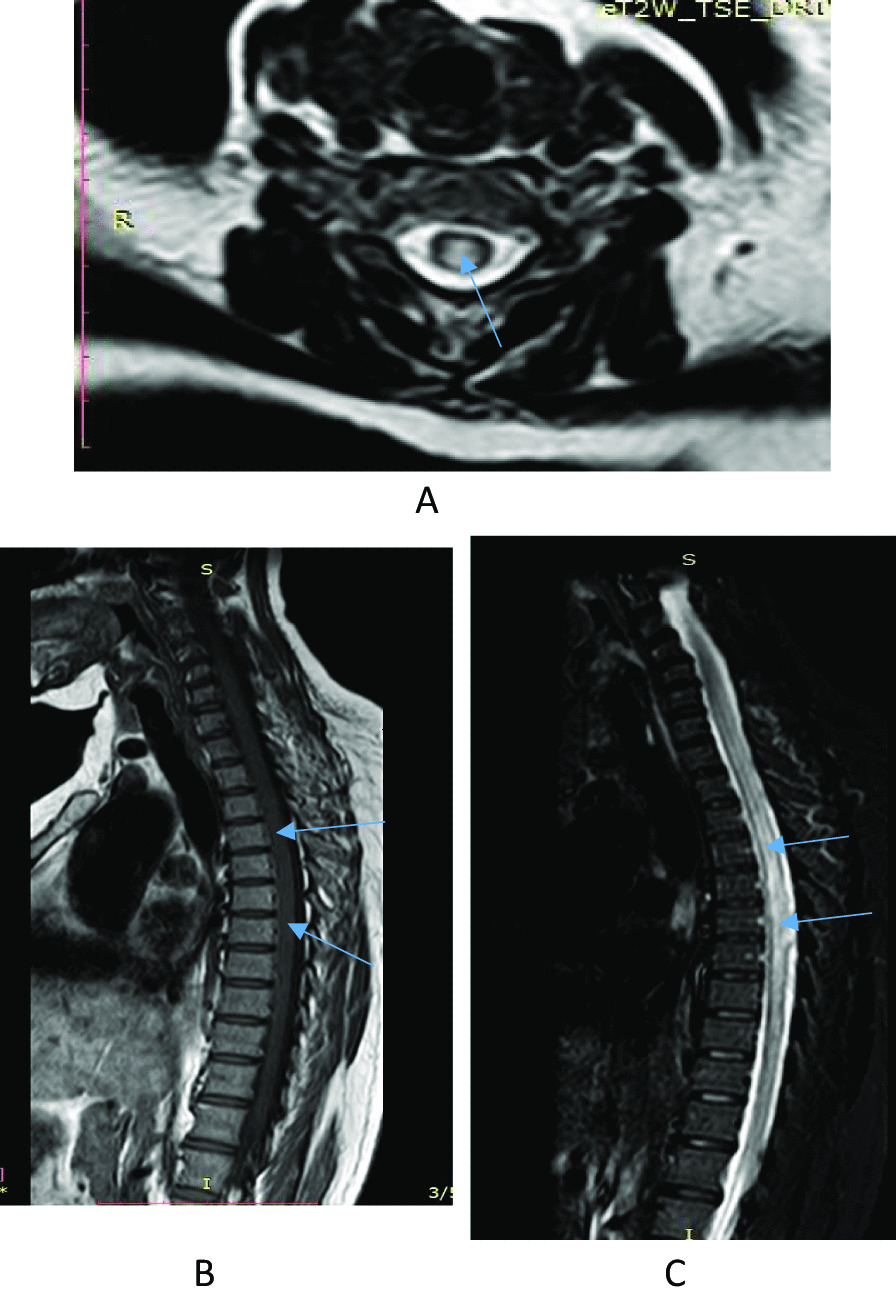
A Magnetic resonance imaging studies of axial T2W image showing hyperintense lesion over the spinal cord. B and C Shows Magnetic resonance imaging studies of Sagittal section showing expansible long segment T1 hypointense, T2 and STIR heterogeneously hyperintense lesion respectively extending from C3 to T12
